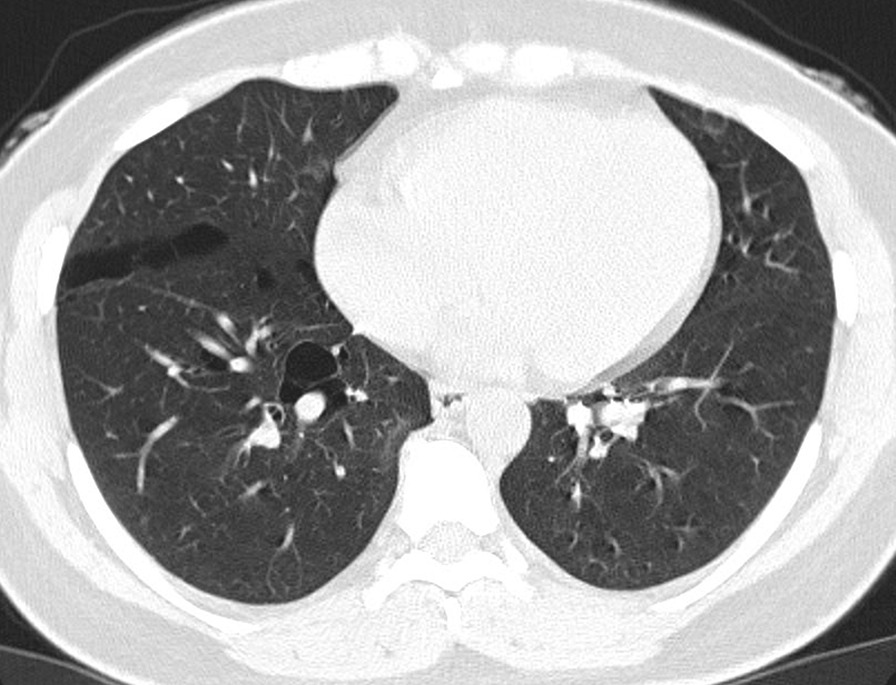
Chest CT images of patients diagnosed with BHD syndrome. Multiple lung cysts ofvarying sizes can be seen. The lung cysts are in the interlobular fissure areas
